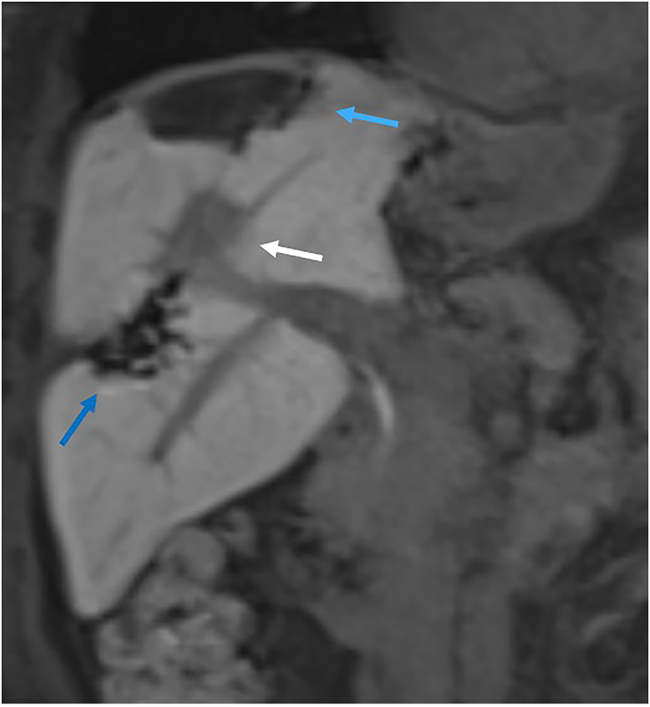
Most recent Primovist-enhanced liver MRI scan, showing contracting SBAR defect (white arrow), and defects from first (dark blue arrow) and second (light blue arrow) liver resections.
